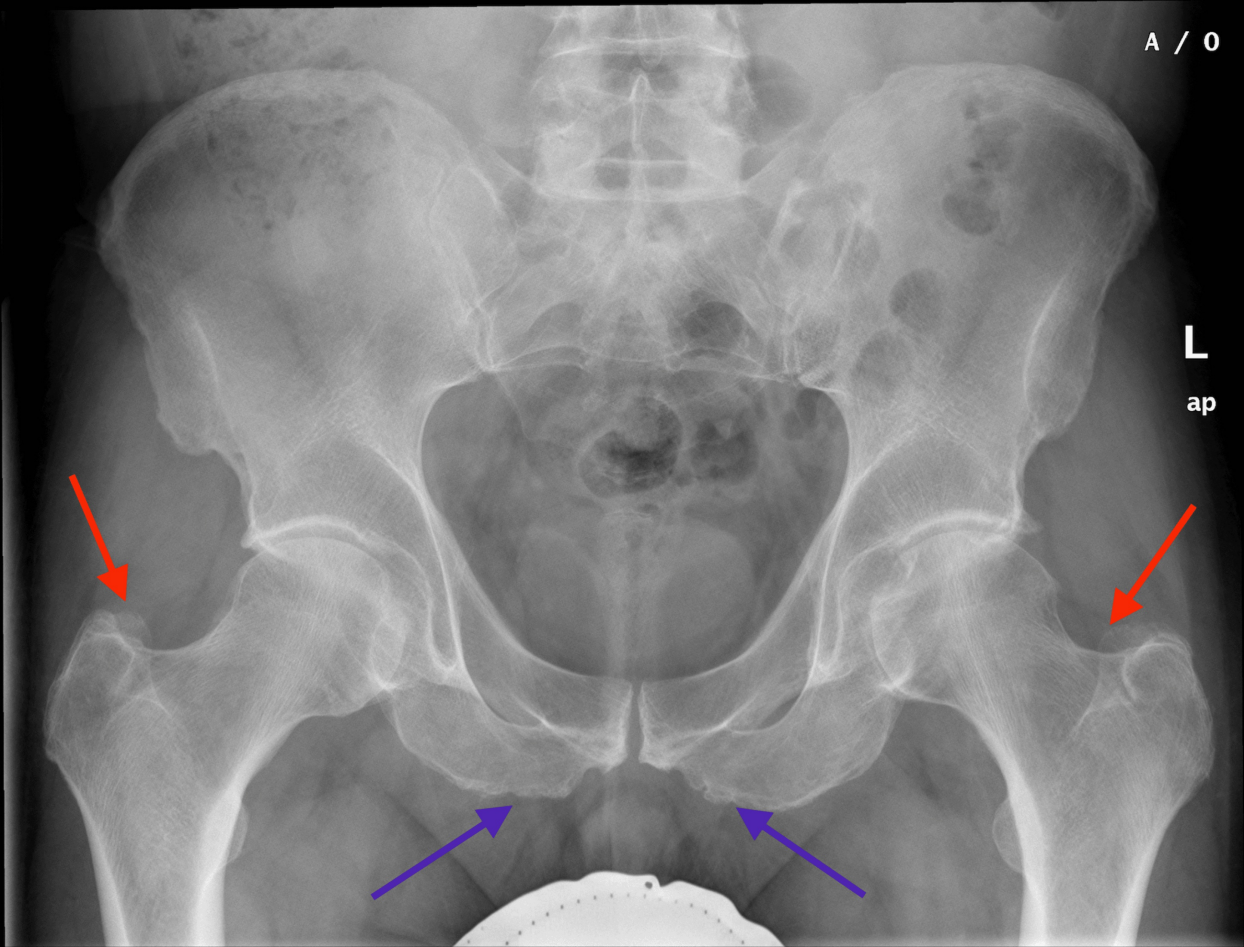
X-ray of the pelvis in the anterior-posterior view Both hip joints showed normal alignment and articular margins. Enthesopathy was seen at bilateral ischial tuberosities (blue arrow) and bilateral supraacetabular prominence (red arrow) was also seen
